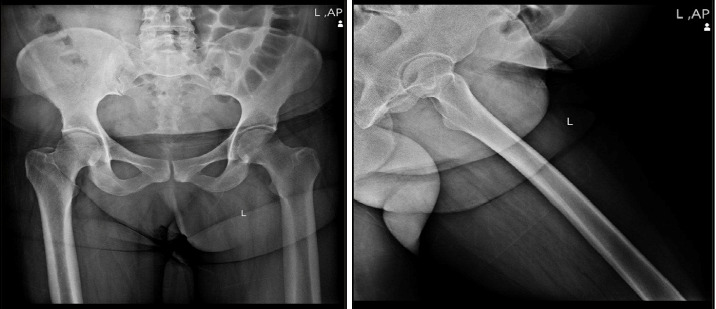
An X-ray examination of the patient, no erosions or bone destruction.
